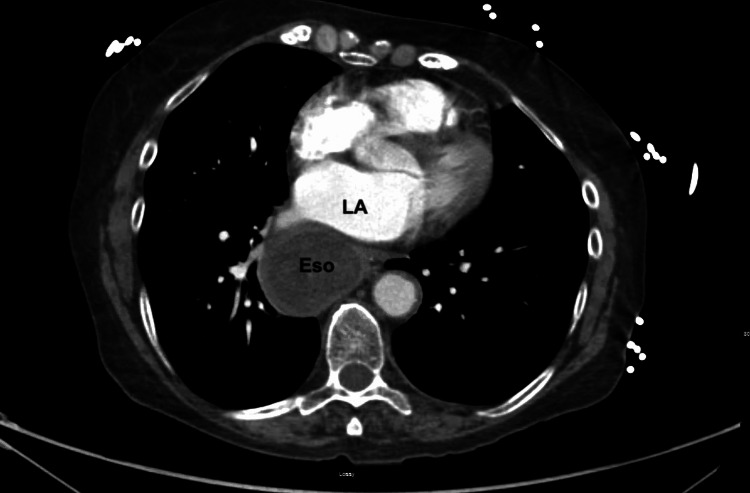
Computed tomography angiography of the chest and abdomen showing markedly dilated esophagus (Eso) against the left atrium (LA) in axial view. Eso: esophagus, LA: left atrium
